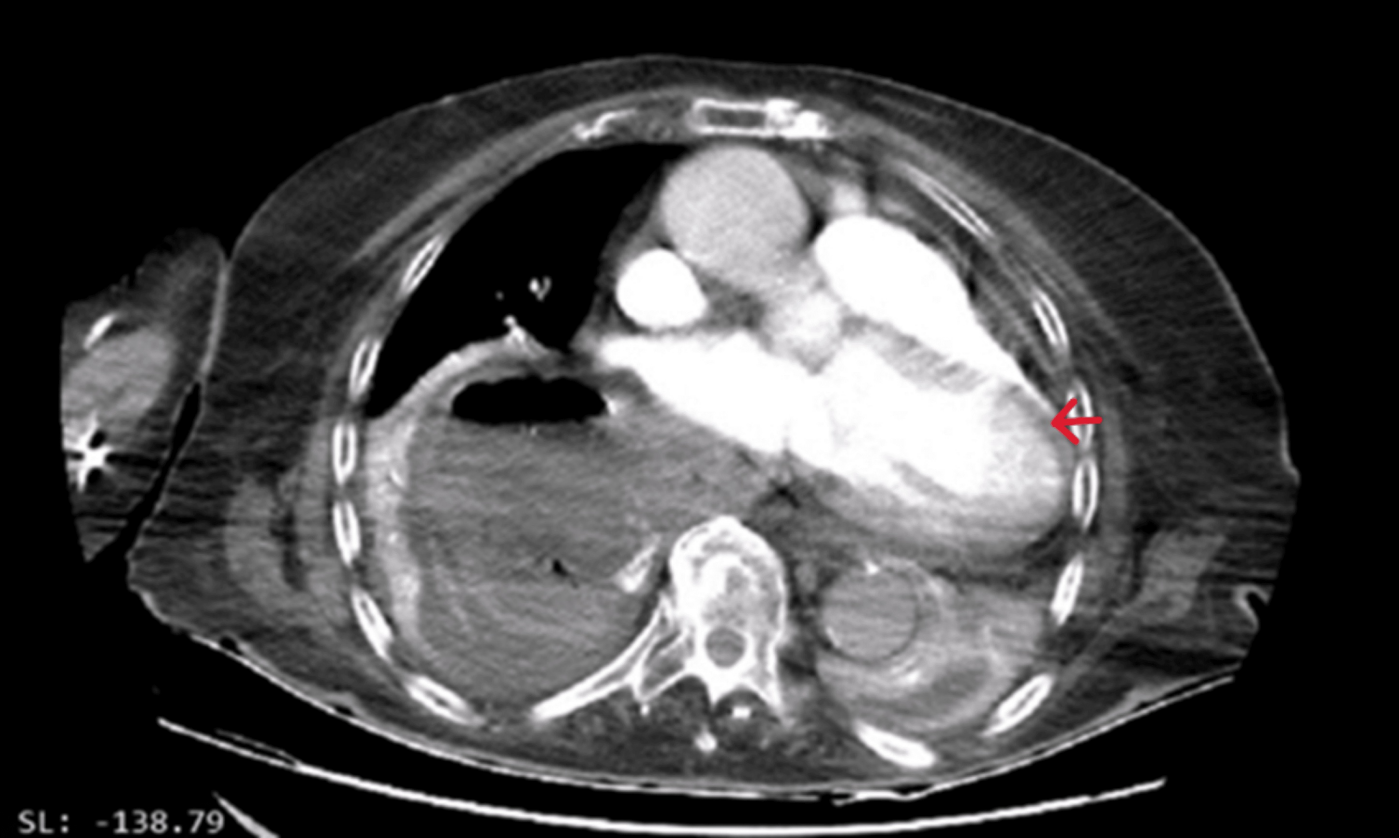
Axial view of Angio CT scan demonstrating a cardiac apex positioned (red arrow) posteriorly and slightly to the right.
